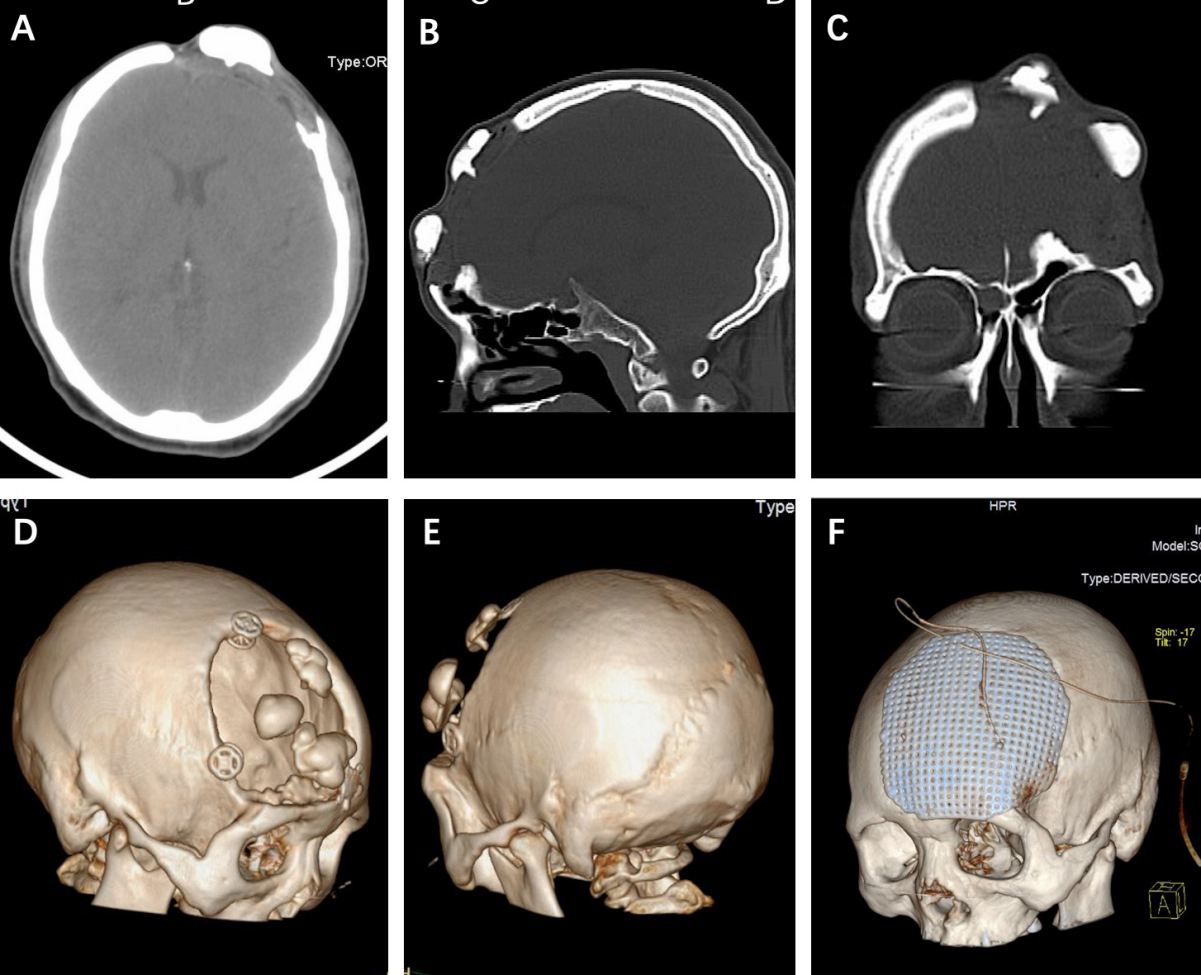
(A-C) Axial, sagittal, and coronary CT scans showed multiple hyperdense lesions without the involvement of bony structure. (D, E) 3D-CT skull reconstruction revealed the multiple lesions located within the cranial defects and internal fixations placed at the edge of the skull defect. (F) The postoperative 3D-CT skull reconstruction of the titanium mesh cranioplasty.
